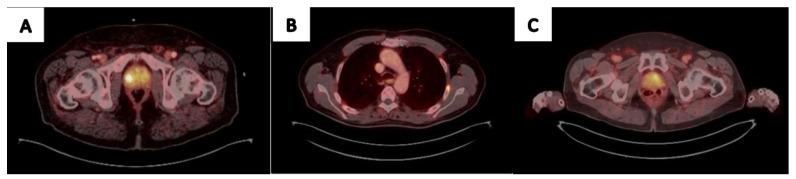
^68^Ga-PSMA Utilization in Real-Life Prostate Cancer Management: (A) 61 yo patient, PSA-6.1mg/ml. PIRAD 3 lesion detected in MRI. ^68^Ga-PSMA PET CT revealed SUVmax 10.6 uptake on the Rt side. Targeted biopsy detected ISUP3 PCa. (B) 67 yo patient, was referred for surgery due to high volume ISUP2 PCa on prostate biopsy. ^68^Ga-PSMA PET CT uptake in the Lt rib without an apparent lesion on CT, concluded as equivocal when revised. PSA remained undetectable for 1 year following surgery. (C) 72 yo patient, PSA 1.05 mg/ml 4.5 years following external beam radiation therapy. ^68^Ga-PSMA PET/CT imaging revealed Lt side local recurrence. Targeted biopsy detected ISUP3 PCa.
